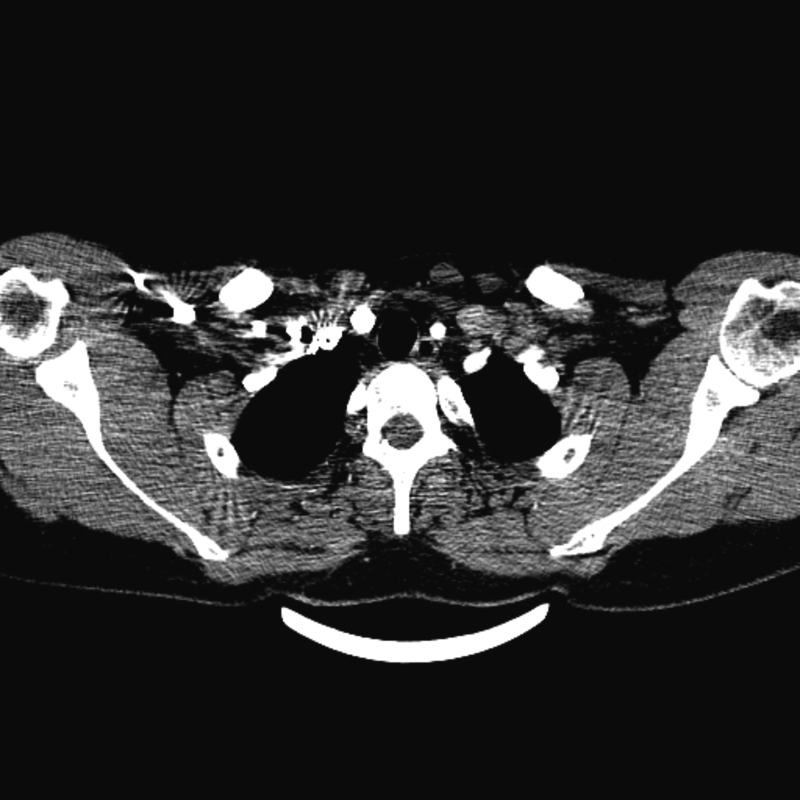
Post-operative contrast-enhanced computed tomography angiogram (CTA).
